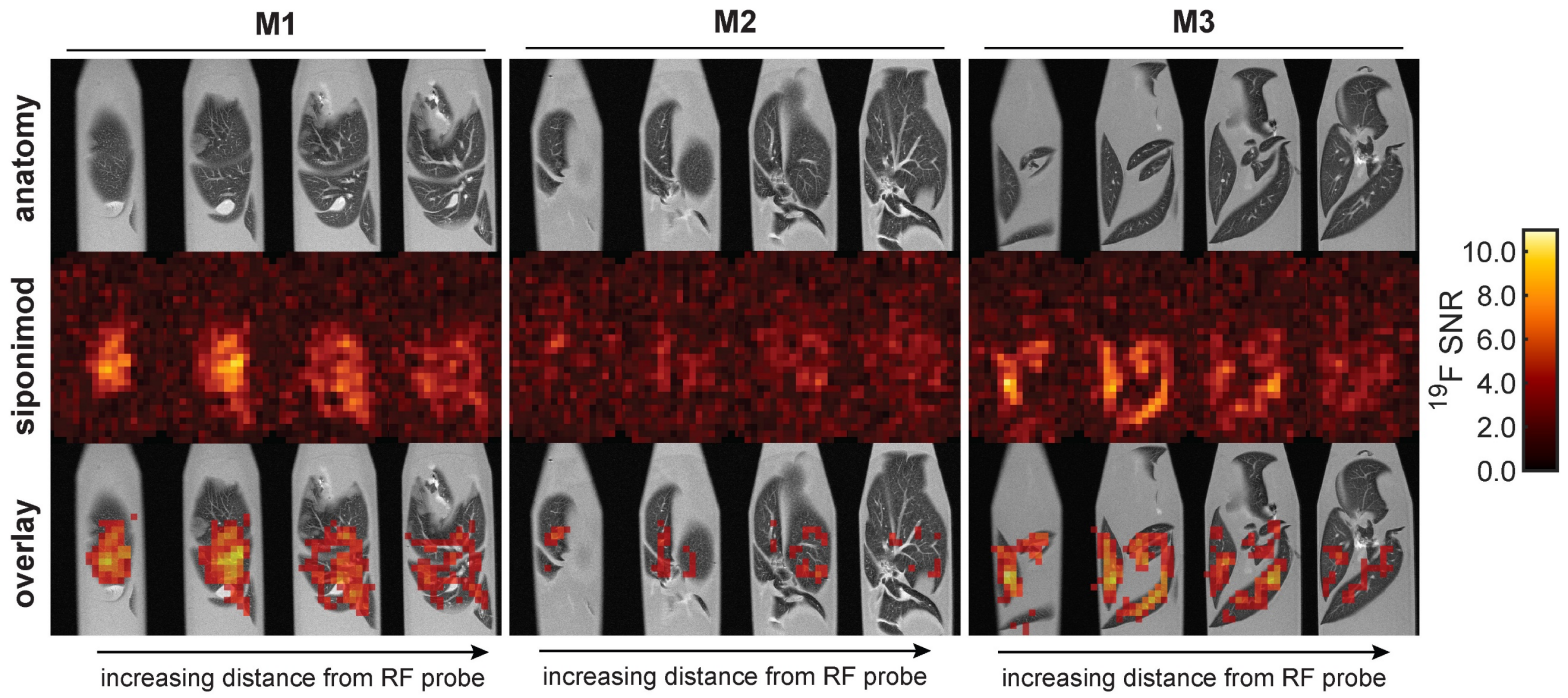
3D UTE ^19^F MRI in *ex vivo* liver tissues with 16 h acquisition time and 0.875 mm isotropic spatial resolution.
